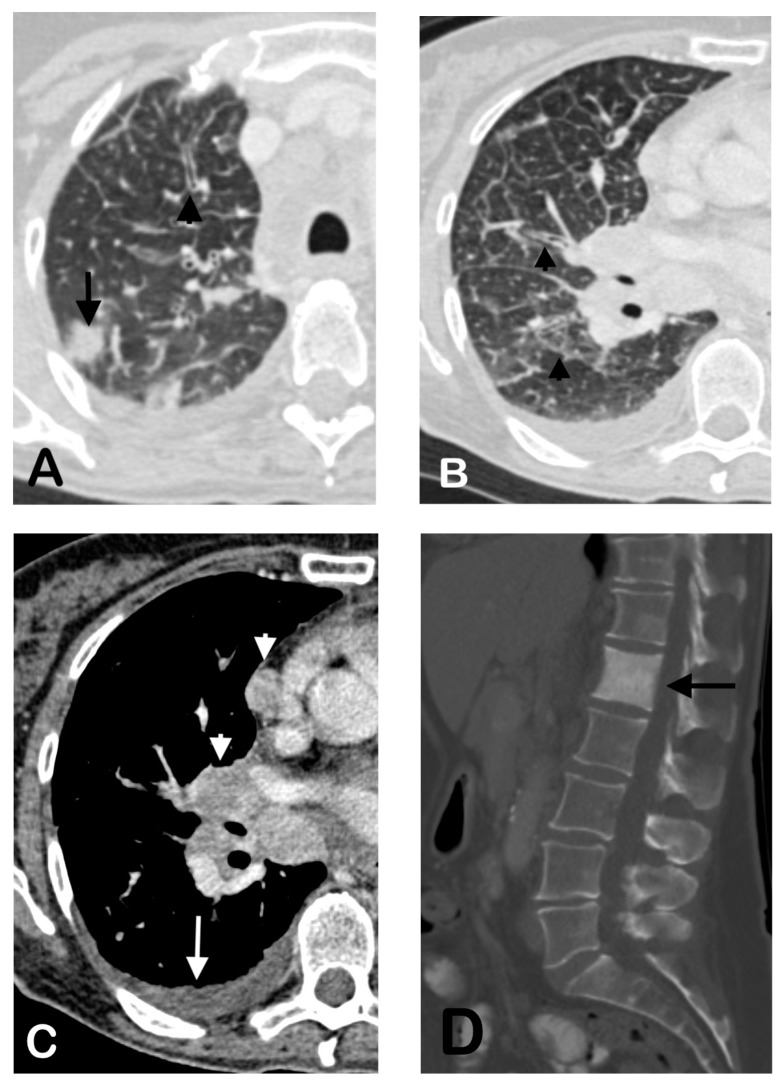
Representative imaging features in a 62-year-old female with *RET*+ NSCLC. Pretreatment CT shows a solid nodule in the peripheral right upper lobe (A, arrow) and associated septal and peribronchial thickening consistent with lymphangitic carcinomatosis (A,B, arrowheads). There was also an associated malignant pleural effusion (C, arrow), mediastinal and hilar lymphadenopathy (C, arrowheads), and a sclerotic osseous metastasis of the first lumbar vertebral body (D, arrow).
